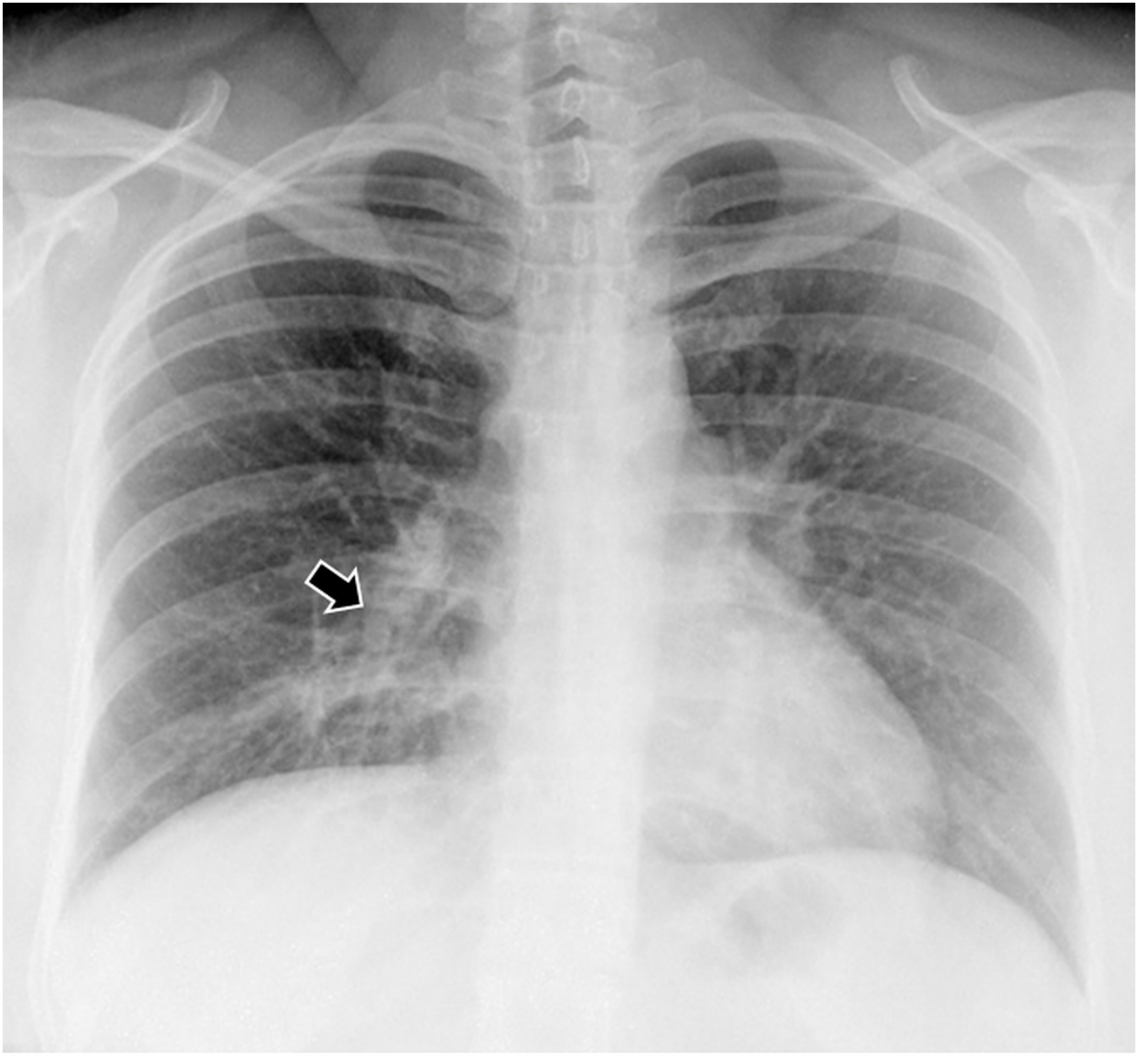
Chest X-ray at admission of a patient with pneumonia by *L*. *anisa*. Radiological signs showed an infiltrate to right lung base (arrow) and a bilateral interstitial pattern.
